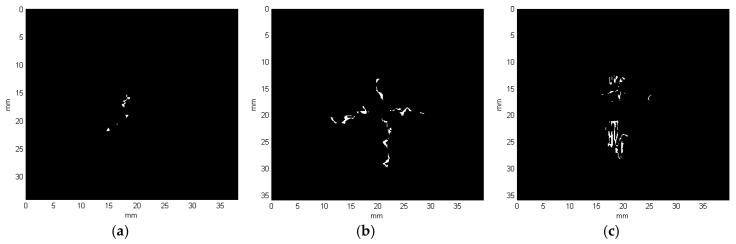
Binary results obtained for the representative X-ray CT slice cases: (a) no. 18; (b) no. 20; (c) no. 99.
